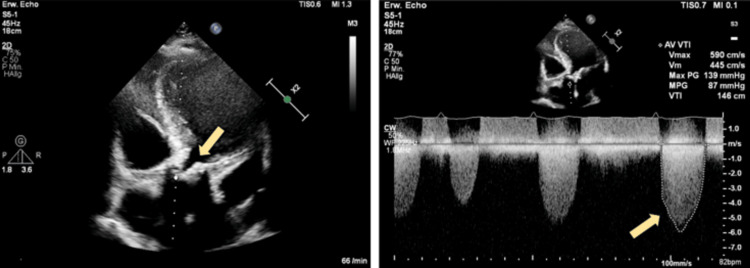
Preprocedural echocardiographic assessment of critical calcific aortic stenosis. (A) Transthoracic echocardiography demonstrating a severely calcified native aortic valve with markedly restricted leaflet mobility and virtually absent leaflet separation (arrow). (B) Continuous-wave Doppler interrogation across the aortic valve showing severe transvalvular obstruction, with a peak velocity of approximately 5.9 m/s and a mean gradient of 90 mmHg (arrow), consistent with critical aortic stenosis.
